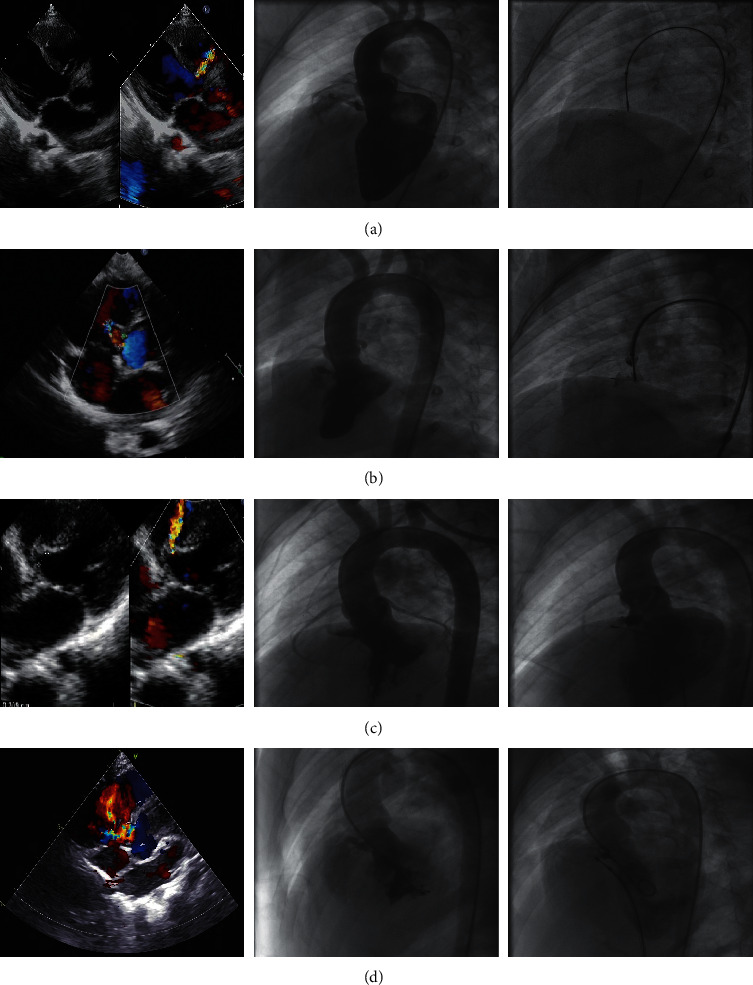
Transthoracic echocardiography (TTE) and angiographic findings considered suitable for ADO II implantation: (a) tubular aneurysm, (b) aneurysm with two constrictions, (c) aneurysm with an elongated conical appearance, and (d) aneurysm with multiple small exits.
